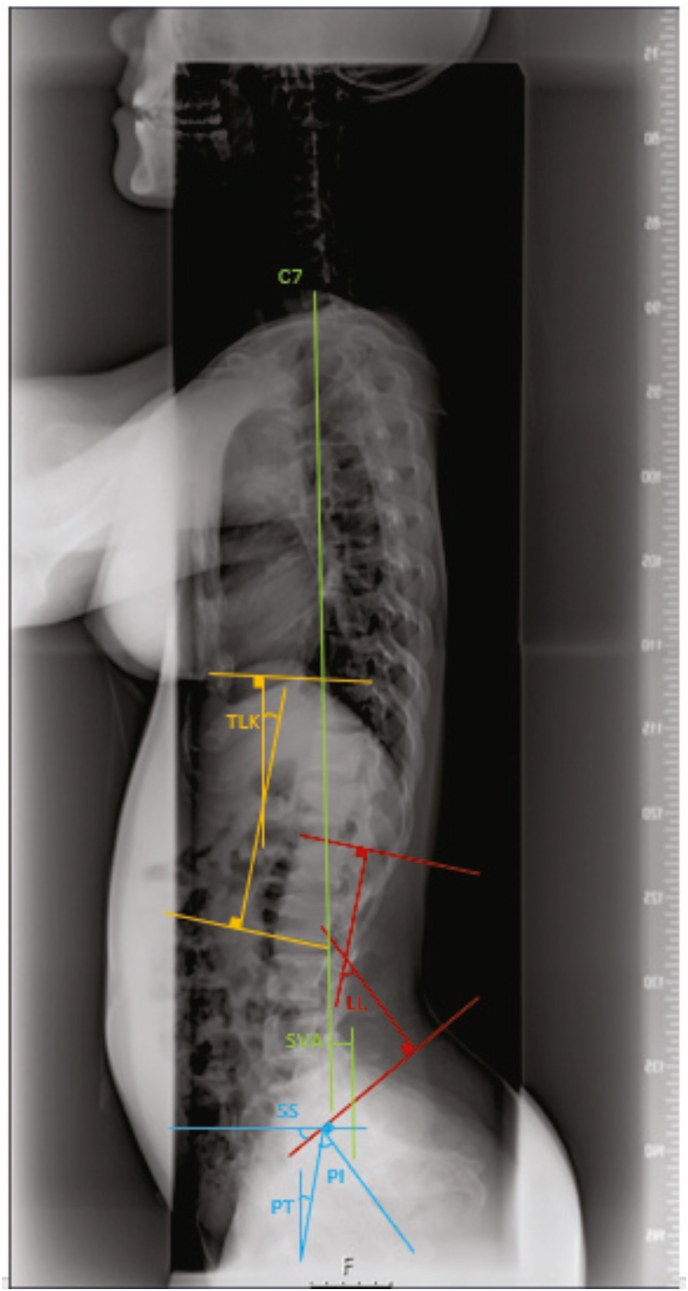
Measurement of sagittal balance parameters. All the assessment are performed on lateral spine radiographs. sagittal vertical axis (SVA) is measured as the horizontal distance between a plumb line from the center of C7 and the plumb line from the posterior-superior corner of the sacrum. Thoracolumbar kyphosis (TLK) is the Cobb angle between the superior endplate of T10 and the inferior endplate of L2. LL is measured as the Cobb angle between the superior endplate of L1 and the superior endplate of S1. Sacral slope (SS) is the angle between the superior endplate of S1 and the horizontal plane. Pelvic incidence (PI) is the angle between the perpendicular line to the sacral plate and the line connecting the midpoint of the sacral plate to the midpoint of the femoral heads. Pelvic tilt (PT) is the angle between the vertical axis arising from the midpoint of the femoral head and the line from the midpoint of the superior endplate of S1 to the center of the femoral head.
